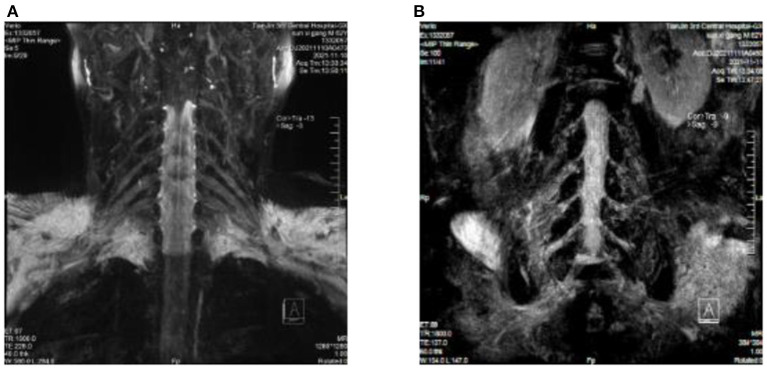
(A) Cervical plexus MRI: fluid attenuation inversion recovery (FLAIR) shows slightly thickened bilateral nerve roots at C4/C5–C6/C7. (B) Lumbosacral plexus MRI: fluid attenuation inversion recovery (FLAIR) shows bilateral nerve root thickening at the L1/L2–L4/L5 intervertebral space, with slightly higher signal intensity, especially at L2/L3–L4/L5.
